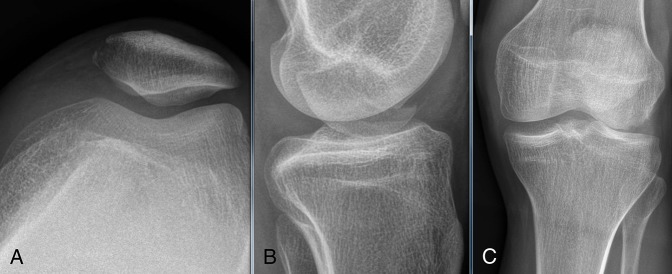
A, Axial radiograph of the left patella. B, Lateral radiograph of the left knee. C, AP radiograph of the knee.
